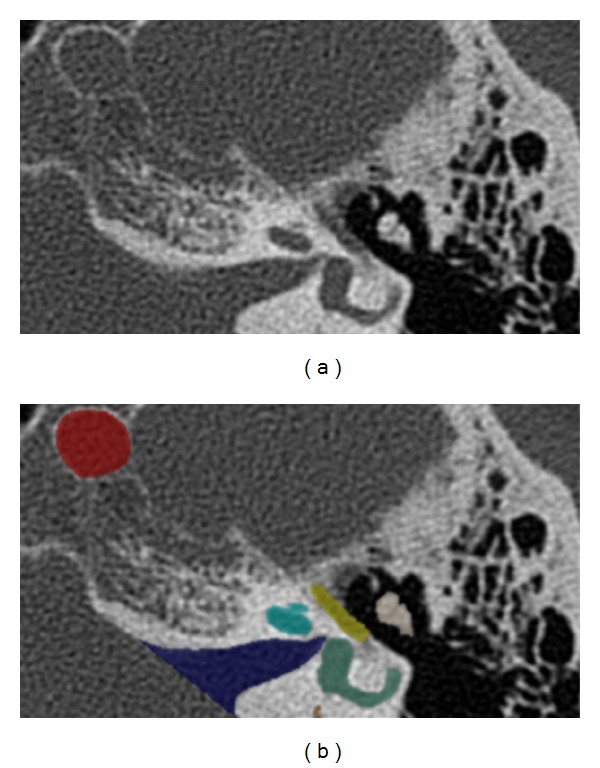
Manual segmentation of the anatomical structures: the left (a) image shows the axial plane of a temporal bone CT scan. The internal carotid artery (red), internal auditory canal (blue), cochlea (teal), labyrinth (green), facial nerve (yellow), and ossicles (beige) were manually segmented in the right (b) image.
